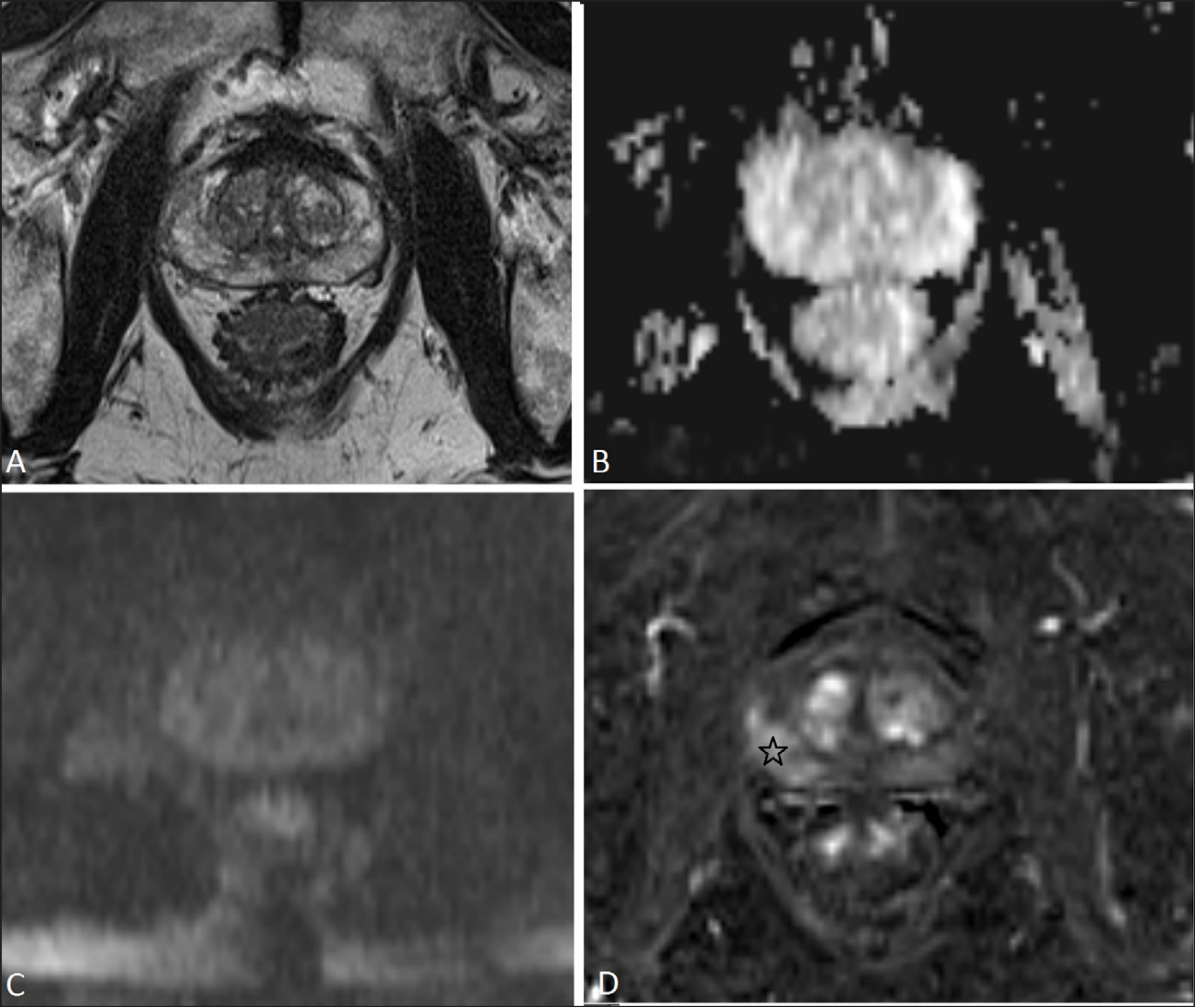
A 66-year-old man with PSA 9.8μg/L. On T2-WI (a) the PZ shows diffuse mild hypointensity, with indistinct margin (PI-RADSv1 score3; PI-RADSv2 score 3). On DWI the PZ shows high ADC values (b) and is isointense on high-b-value image (c) (PI-RADSv1 score 1; PI-RADSv2 score 1). On DCE there is a focal enhancing lesion posterolateral on the right side in the PZ (black star) (PI-RADSv1 score 4; PI-RADSv2 positive). With the PI-RADSv1 scoring system, this patient was assigned an overall assessment score 4, based on a subjective overall impression of the findings in all modalities. With the PI-RADSv2 scoring system this patient was assigned an overall assessment score 1 because DWI was scored 1 and this is the dominant modality in PZ. Prostate biopsy followed by radical prostatectomy in this patient showed a Gleason 3+4 prostate cancer on the right side in the PZ.
